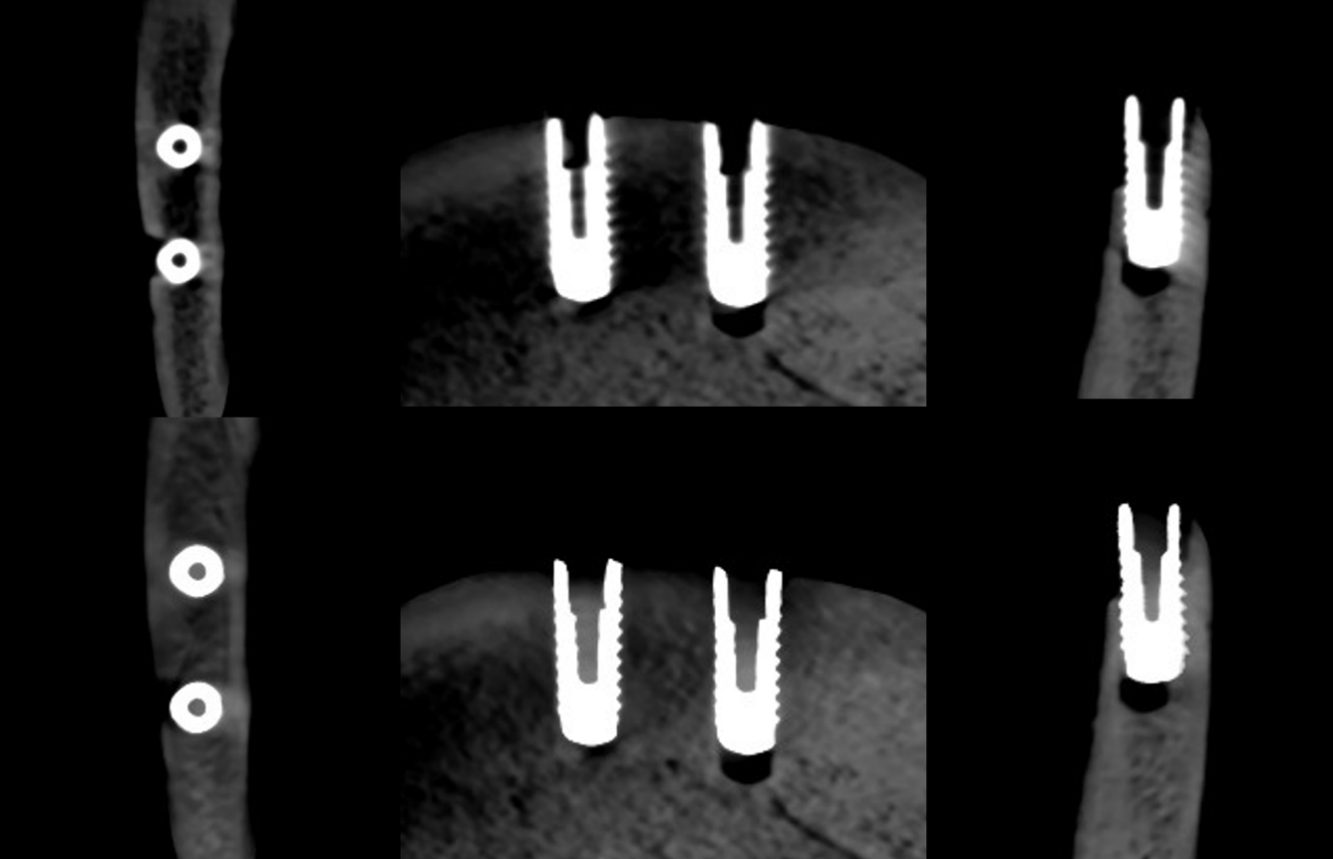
Axial, sagittal, and coronal slices of the CBCT image of the titanium implants with the imaging parameters 3. Above no ARA and below ARA 3. The defect is present in the images and the bone structure between the implants is smooth with ARA 3. The spiral structure is seen in detail. ARA, artifact reduction algorithm; CBCT, cone beam CT.
